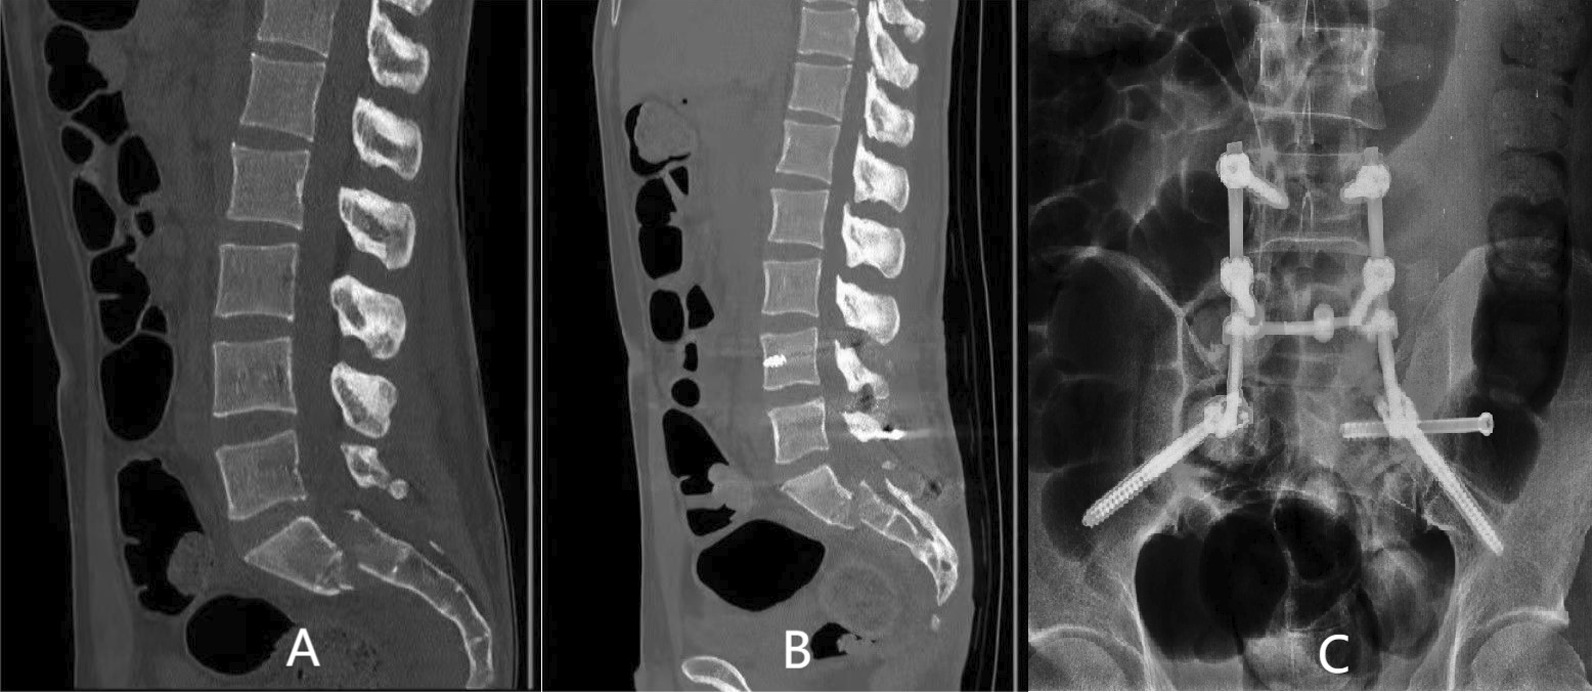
Case 12. A 16-year-old man who was suffered from fall injury from height underwent spinopelvic fixation and S1 sacroiliac screw fixation for a 301SPD type Ic fracture. A Preoperative CT scan of the sacrum in the midline sagittal plane shows anterior flexion displacement. B Postoperative CT scan midline sagittal image demonstrates good reduction of fracture. C The radiological examination image shows that the fracture was reduced and fixed satisfactorily
